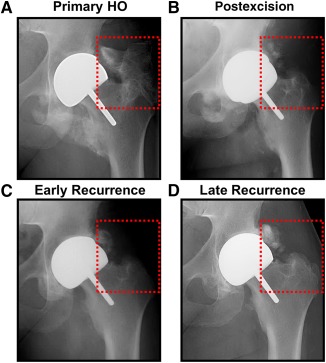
Clinical scenario of recurrent HO in a patient with total hip arthroplasty. (A): Primary HO in total hip arthroplasty patient (red square). (B): Postexcision radiograph showing substantial reduction in HO with some residual lesions. (C): Early evidence of recurrence after excision of HO. (D): Recurrent HO lesion 6 months after surgery at excision site. Abbreviation: HO, heterotopic ossification.
